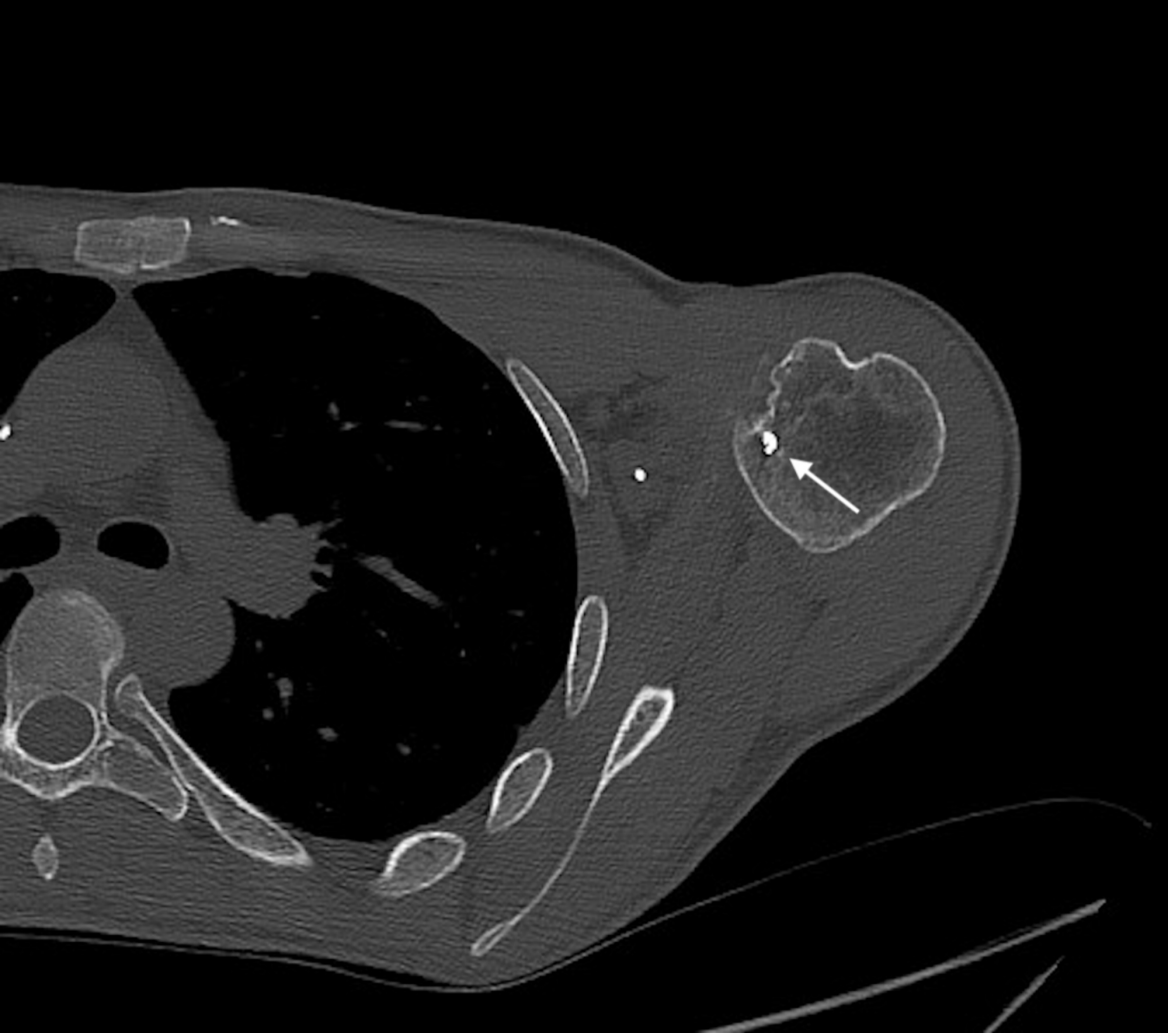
Axial CT shoulder showing an anterior humeral head suture anchor with partially visualized reverse Hill-Sachs deformity CT: computed tomography
